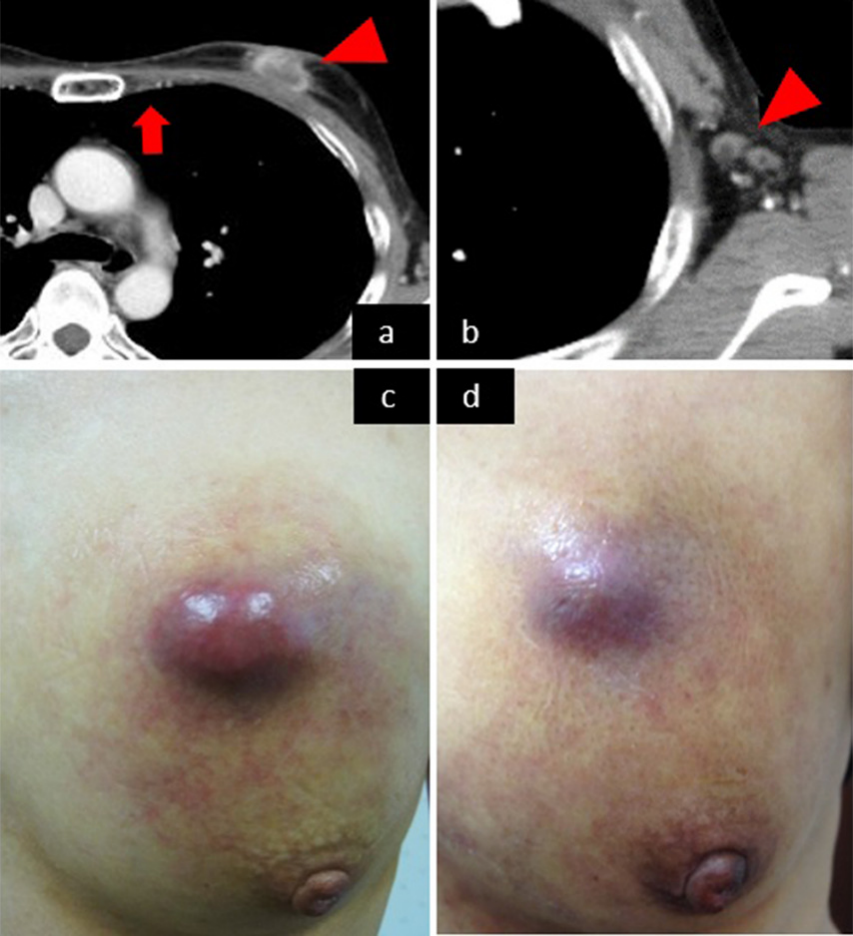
CT images and photographs demonstrating the effect of treatment. The primary lesion (arrowhead in a) and axillary lymph node (arrowhead in b) were reduced in size and the parasternal lymph node (arrow in a) disappeared after the third session. The skin lesion before treatment was reddish, oedematous and tense (c), which improved after the first treatment session (d).
